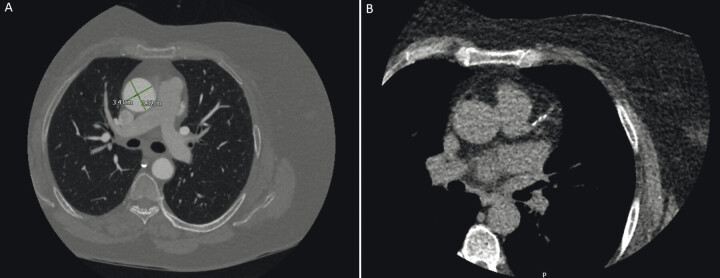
Computed tomography imaging used for procedural planning. (
